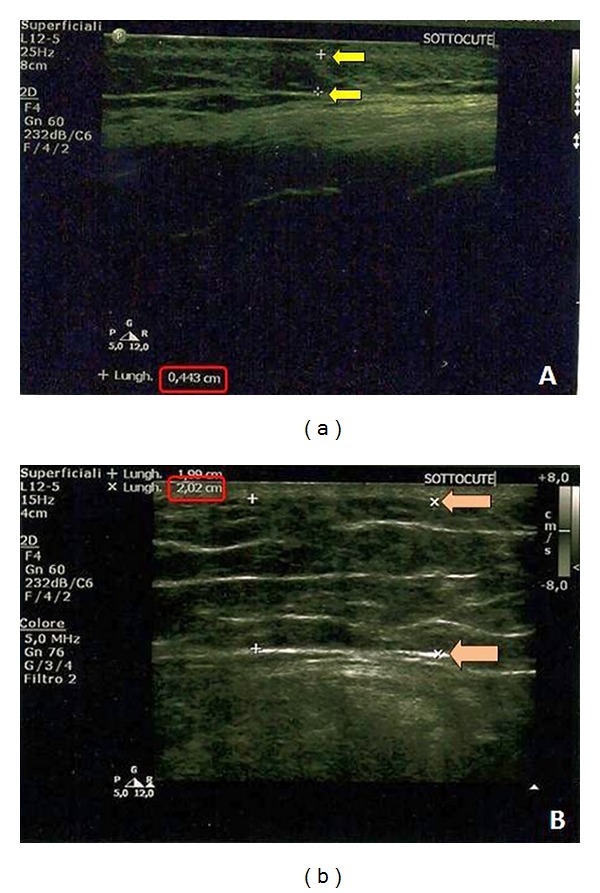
(a) Preoperative subcutaneous tissue ultrasonography; (b) 3-month postoperative subcutaneous tissue ultrasonography. Shown by the arrows is the increase of the subcutaneous thickness.
